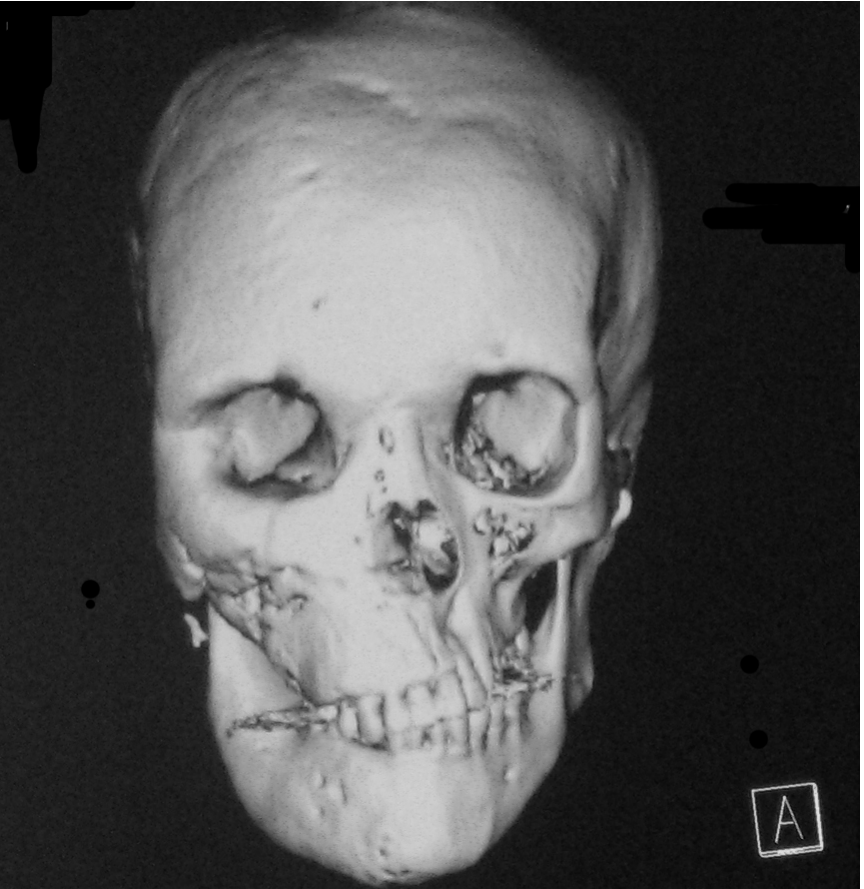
Reconstructed three-dimensional CT image shows lion face appearance due to asymmetrical involvement and enlargement of the bones of the face and the skull.
